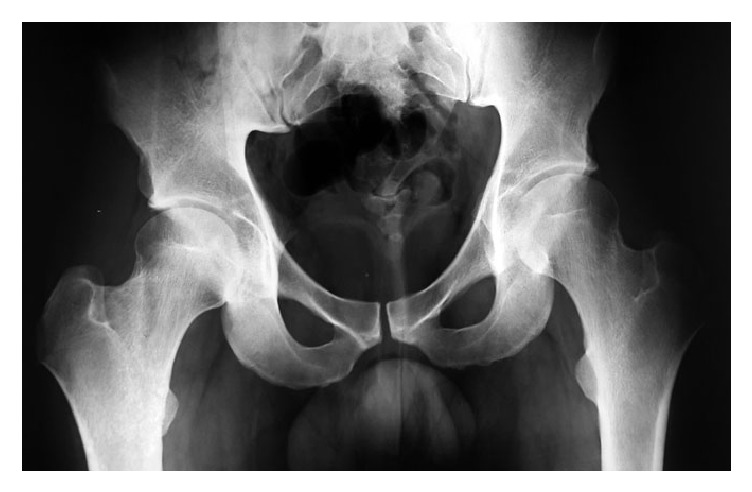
AP view radiograph of the pelvis revealing opacity in the left acetabular fossa.
